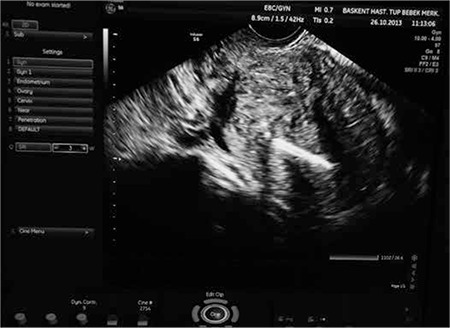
Ultrasonographic image of intrauterine device in the uterine cavity and the neocervix extending in the lower part of uterus
